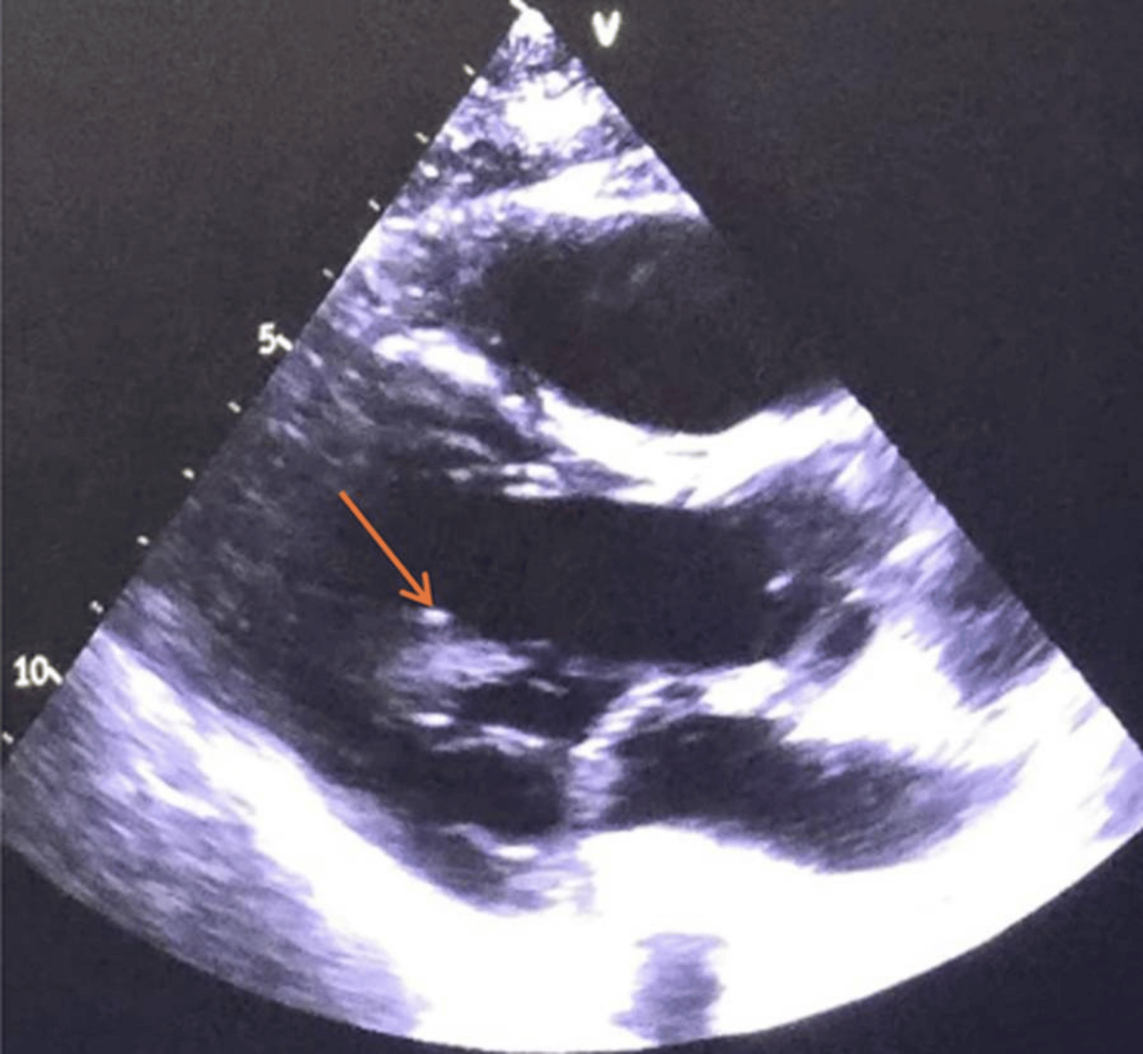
Parasternal long-axis (PLAX) view on transthoracic echocardiogram The echocardiogram image demonstrates voluminous, irregular, and echogenic masses (vegetations) attached to the atrial surface of the mitral valve leaflets and the aortic valve apparatus.
